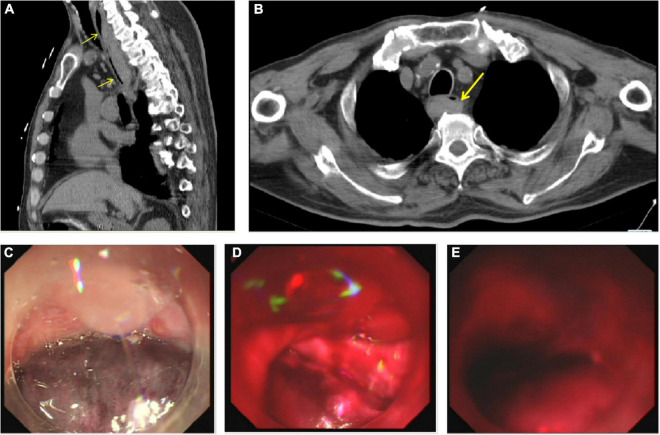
(A) Sagittal chest computed tomography (CT) image reconstruction showed the hematoma extending from the entrance of the esophagus to the gastroesophageal junction (yellow arrows). (B) Axial chest CT image reconstruction showed the hematoma occupying more than half of the lumen (yellow arrow). (C) Endoscopy revealed esophageal mucosal exfoliation in the upper third of the esophagus, (D) middle third of the esophagus, and (E) lower third of the esophagus.
